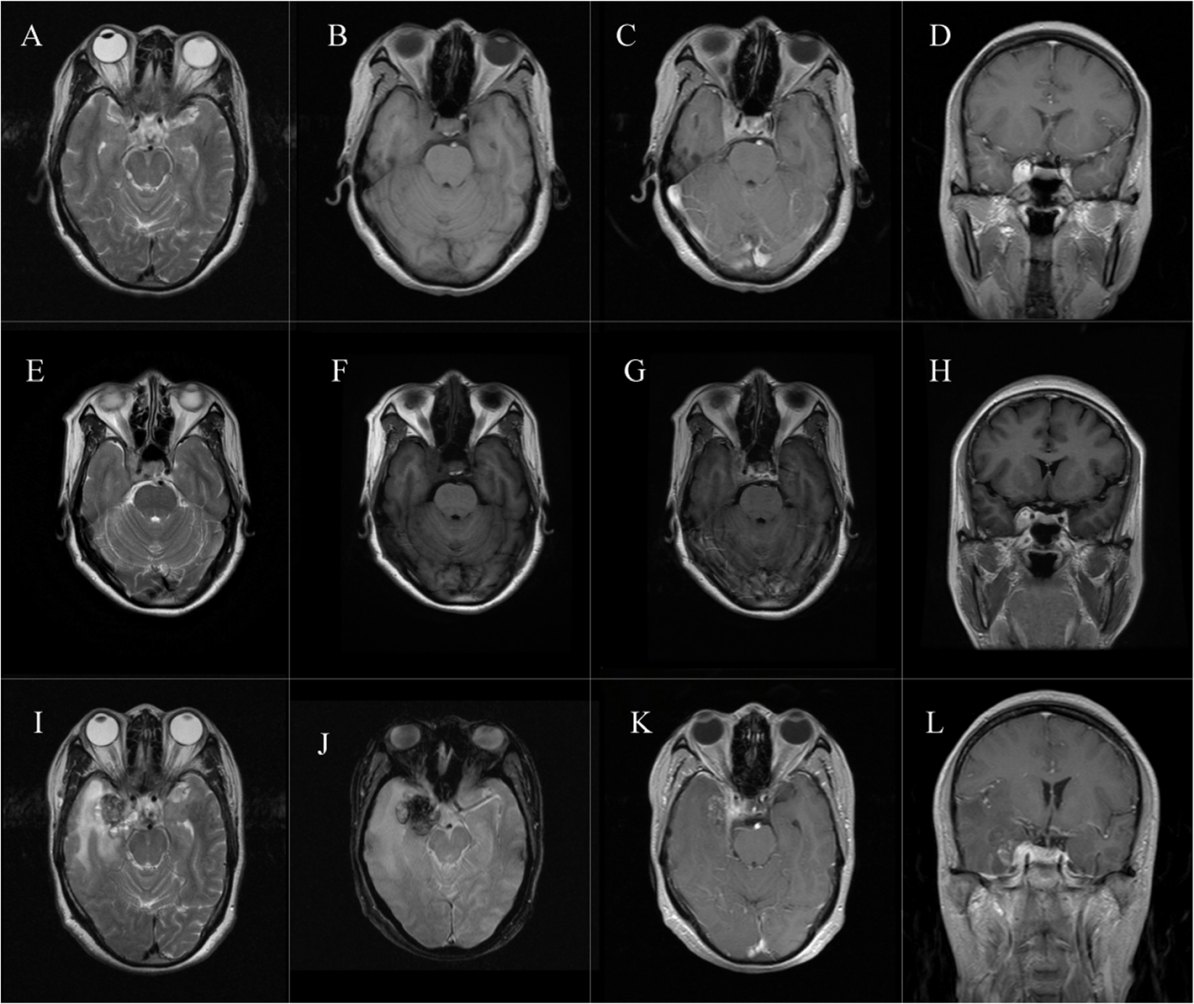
MR images before surgery: MR images before SRS show irregular nidus with abnormal signal in the right parasellar region(a, b, c, d).)MR images 8 months after SRS show size of CSM is similar to that before treatment (e, f, g, h). MR images after admission show that the size of CSM was larger than that of 8 months after SRS. Another lesion appears on the right temporal lobe (i, j, k, l)
